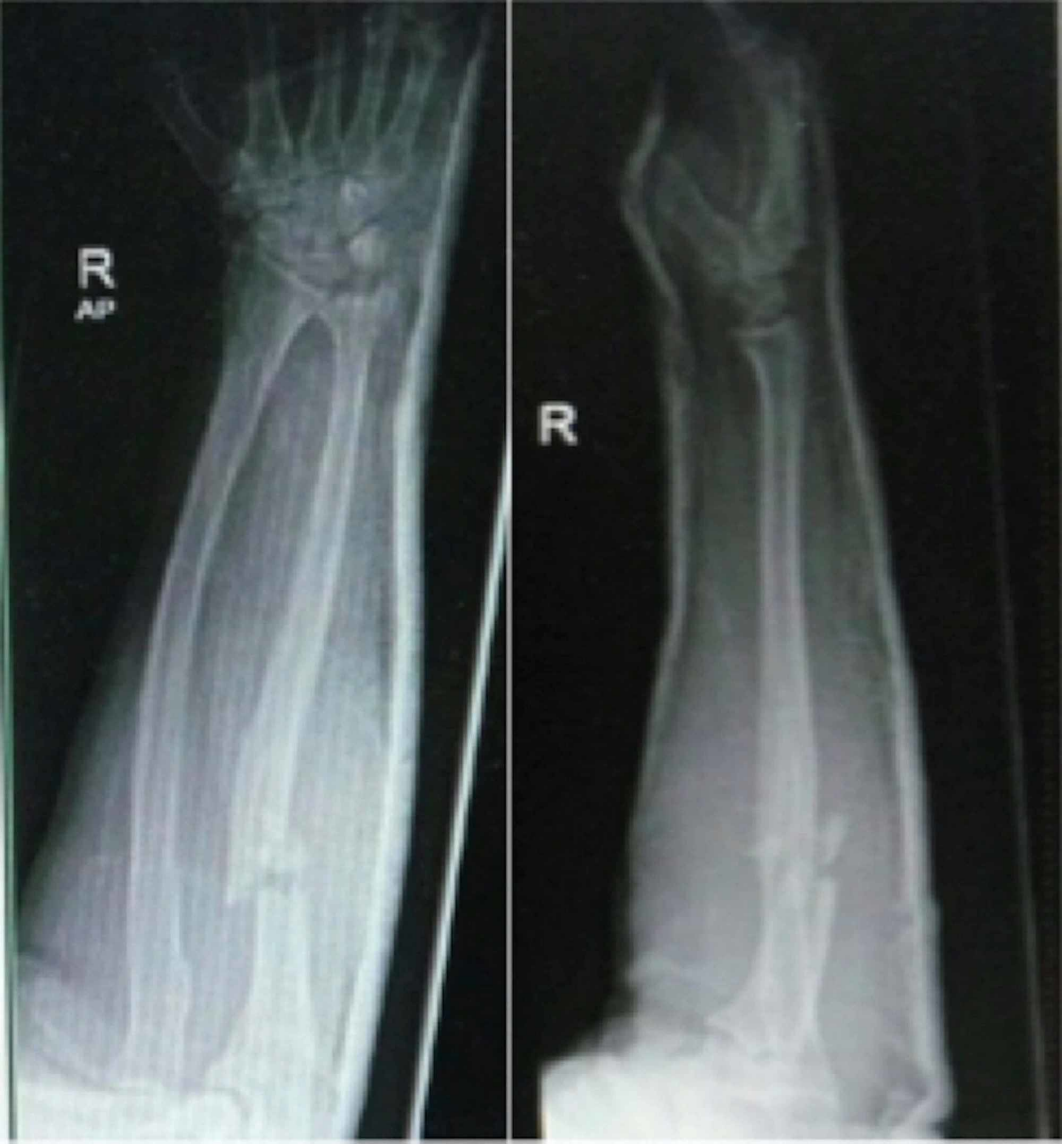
Radiograph showing Monteggia fracture of right forearm (AP and lateral view).
